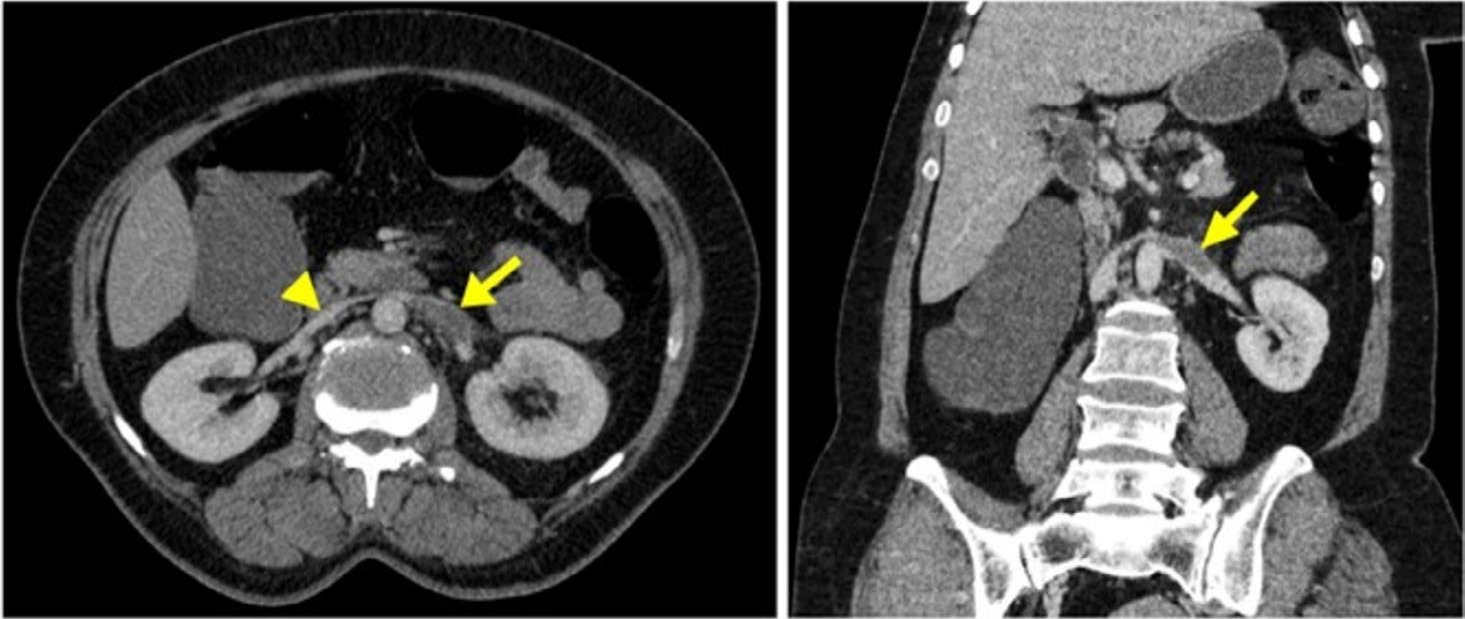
Left: A contrast‐enhanced CT scan (CECT) in the axial plane shows a dilated left renal vein with a hypodense filling defect, which is called thrombosis (*arrow*). Note the right renal vein with normal diameter and completely contrast‐filled (*arrowhead*). Right: In the coronal view, the arrow also indicates left renal vein thrombosis.
